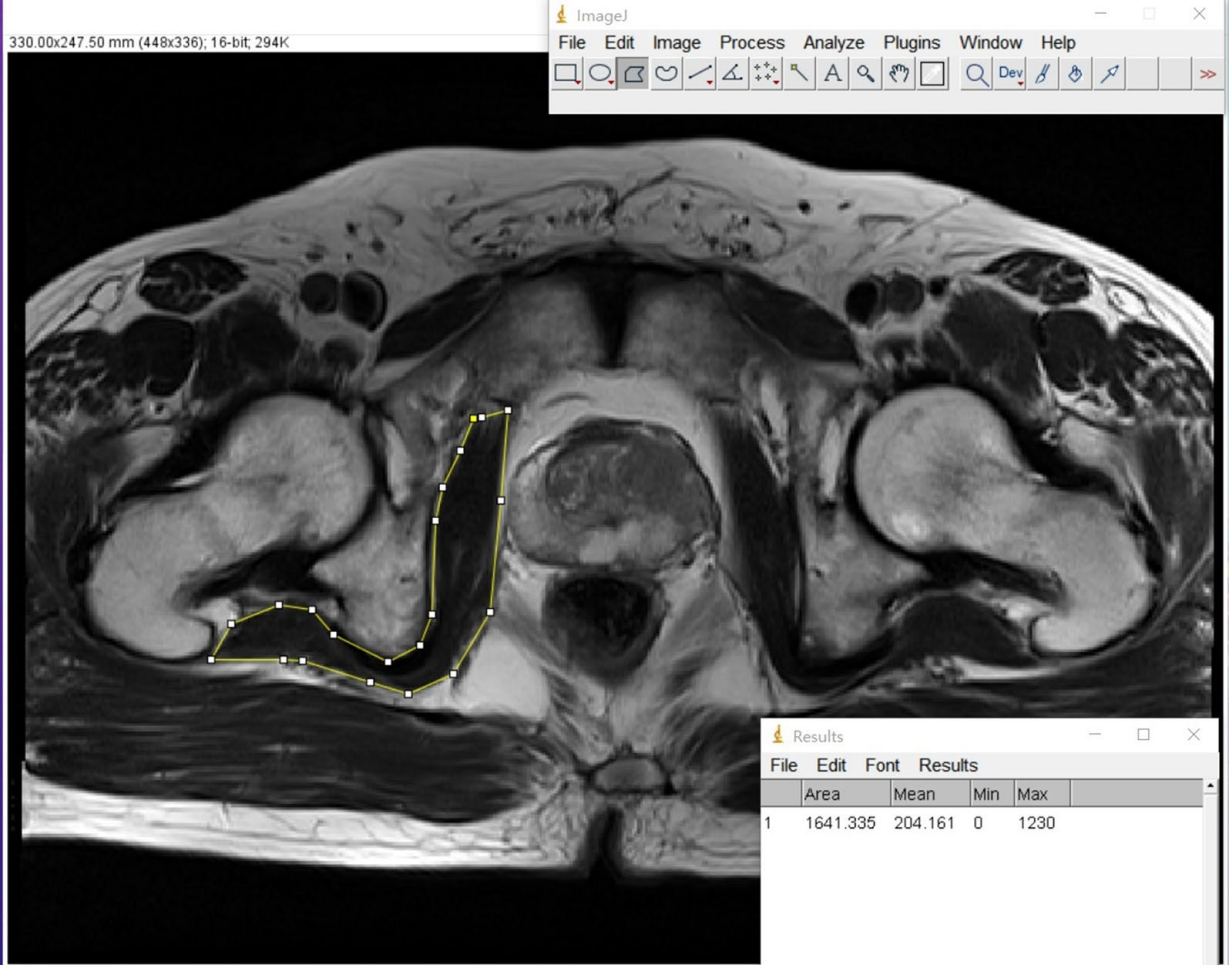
The measuring methods of internal obturator muscle width in the pelvis MRI for IOM mass index
